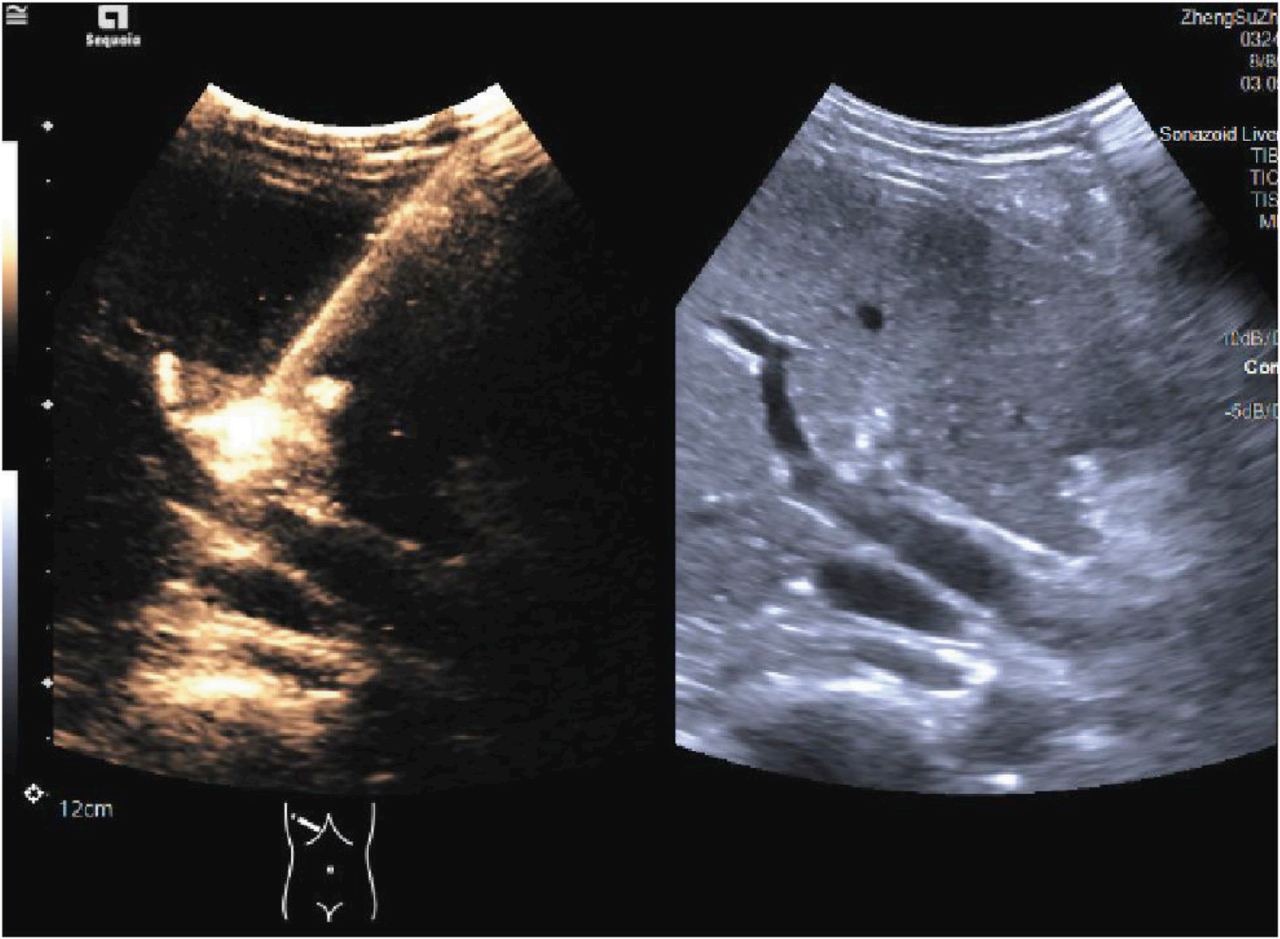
Ultrasound-guided HHNB. HHNB: hepatic hilar nerve block.
